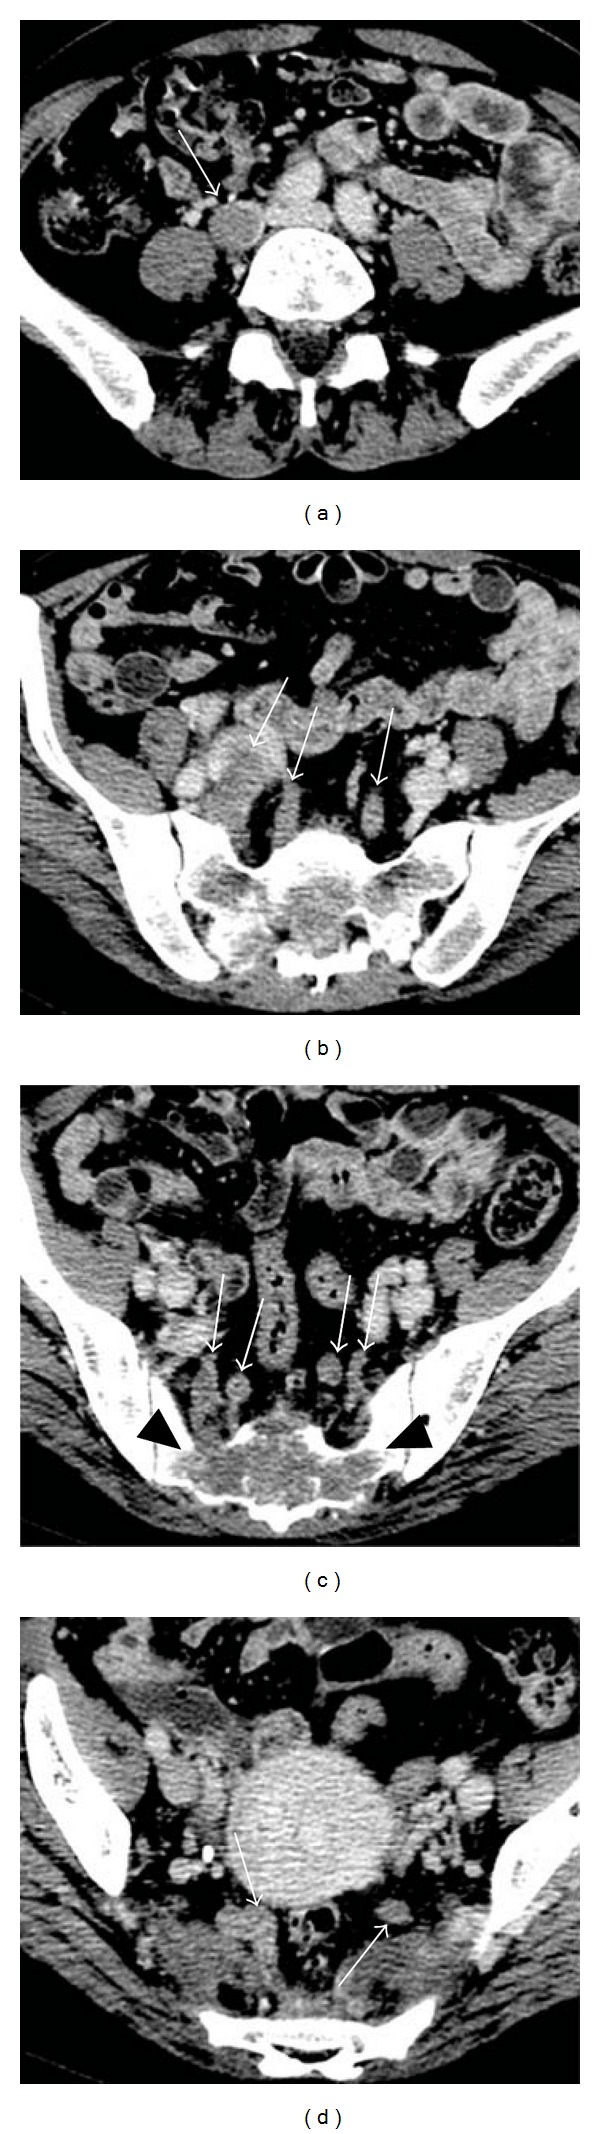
Contrast-enhanced CT demonstrated intraluminal filling defects in the right common iliac vein, both internal iliac veins, and their branches ((a)–(d)) (white arrows). A lytic lesion in the sacrum was also noted (c) (black arrowheads) and continuity between the sacral lesion and the filling defect in the branch of the right internal iliac vein was indicated.
